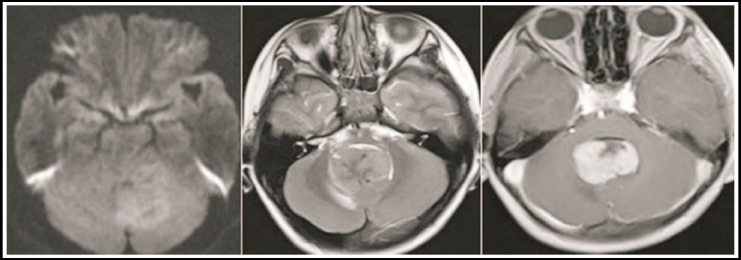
MRI image of 9-year-old Burkitt lymphoma.
